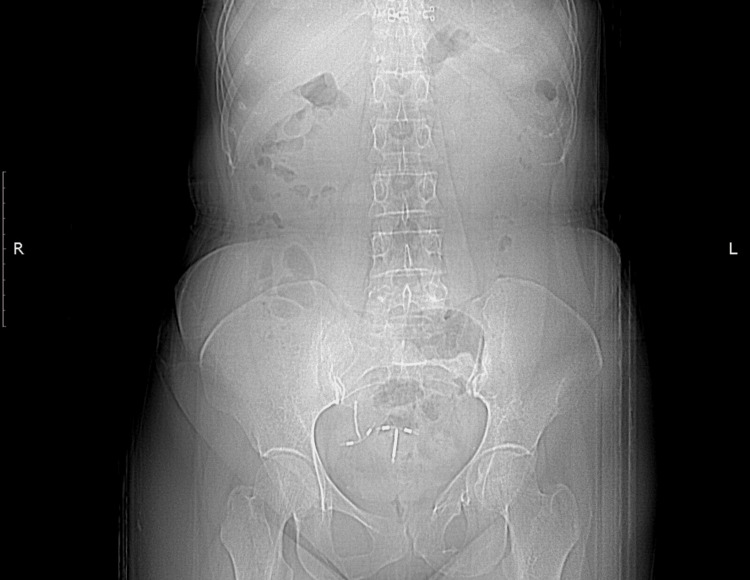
On abdominopelvic X-ray, there was an appearance consistent with two intrauterine devices, one in the region consistent with the course of the right distal ureter and the other in the normal localisation.
